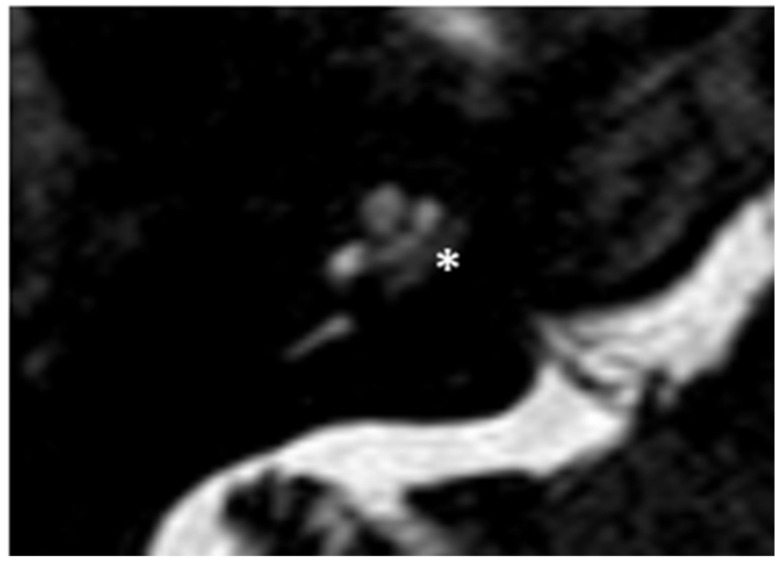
MR image of patient #1 with osteogenesis Imperfecta. MR cisternography showing the fluid-signal in the newly formed cavity (white asterisk).
